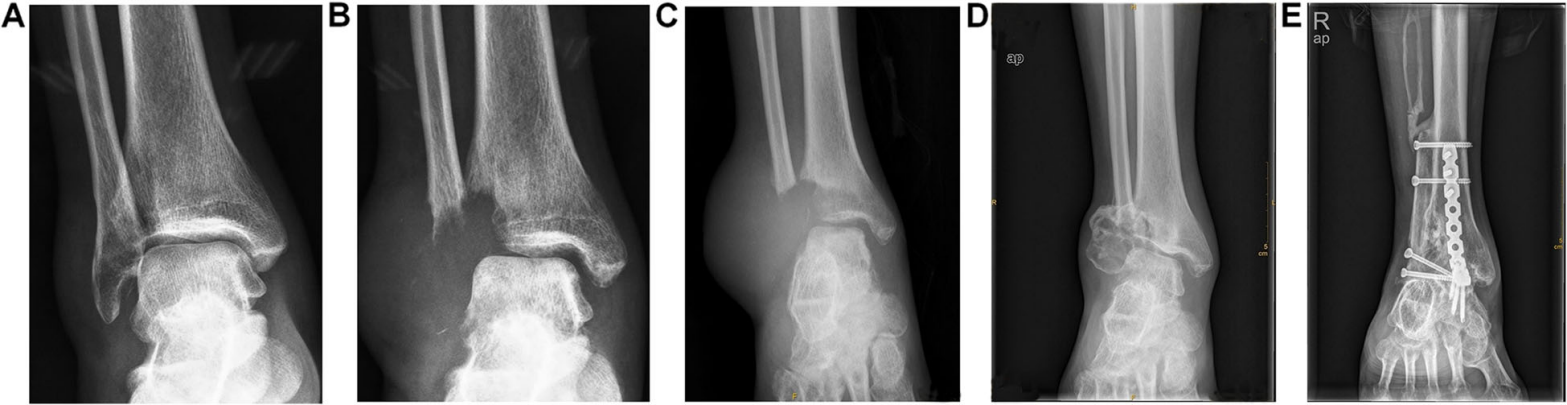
Radiographs images of the right lateral malleolus over the course of treatment. a Anteroposterior radiograph of the patient at the first visit after trauma; b two months after the trauma, radiograph shows massive osteolysis and obvious soft-tissue edema at right distal tibiofibular; c one month after medical treatment, radiograph shows arrest of the osteolysis process; d seven months after medical treatment, radiograph shows shrinkage of the lesion and ossifications and sclerosis; e eight years after reconstruction surgery, radiograph shows rigid internal fixation with primary union without disease progression
